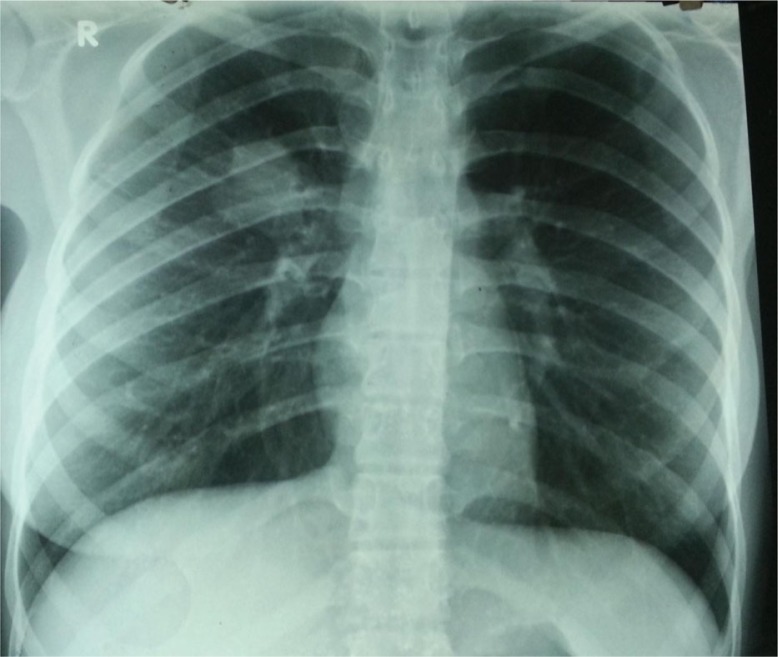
Initial chest radiograph.
